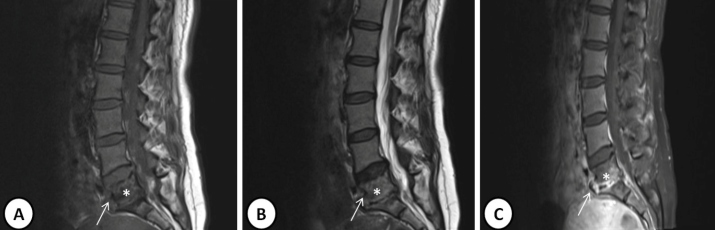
Post-treatment images showing narrowing in the L5-S1 discs in (A) T1-weighted, (B) T2-weighted (B), and post-contrast images (C). The white arrow showsdecreased pathological signals in the corners of the L5-S1 corpus exhibiting extension to the anterior epidural fat. (L5- *).
